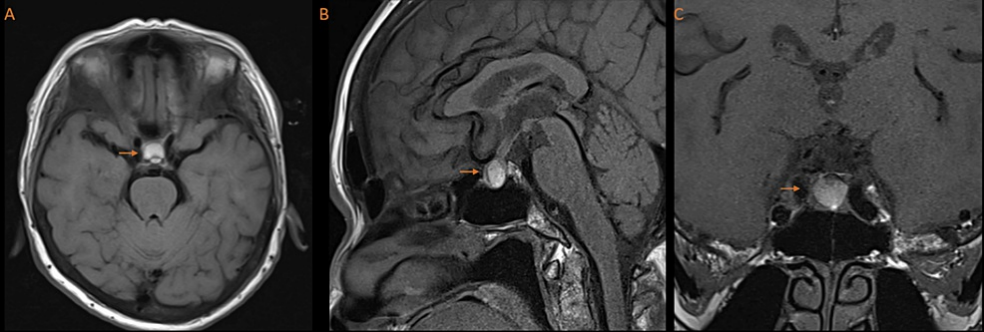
Axial (A), sagittal (B), and coronal (C) T1-weighted images of sellar/suprasellar oval-shaped homogenous hyperintense mass (arrows), effacing the suprasellar cistern, no cavernous sinus invasion, no definite optic chiasm compression.
